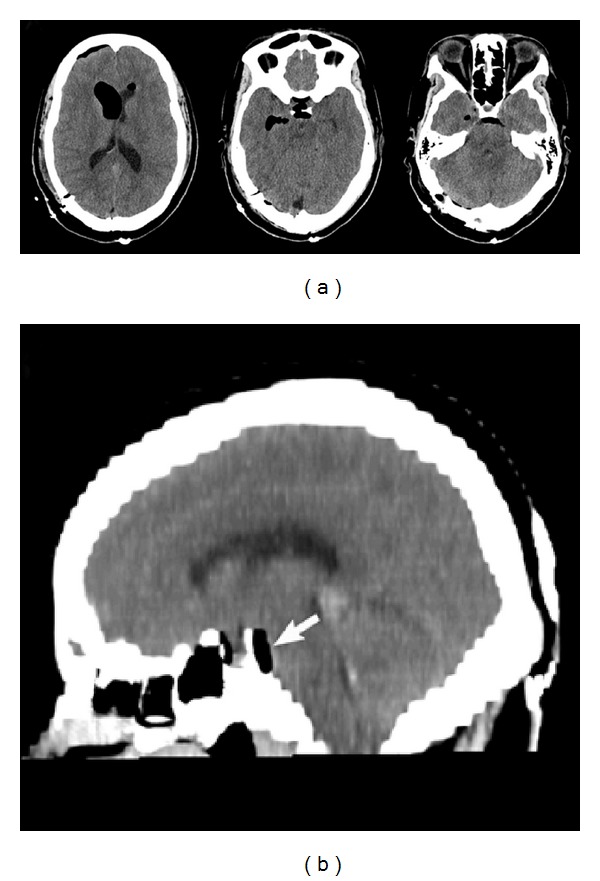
Immediate postoperative CT scan. Postoperative changes following resection of pineal region cystic lesion are evident in conjunction with significant pneumocephalus. Intracranial air is seen diffusely in the subarachnoid and ventricular spaces (a). A significant amount of air is also noted in the interpeduncular and prepontine cisterns. The midsagittal reconstructed image highlights the interpeduncular air (arrow; (b)).
